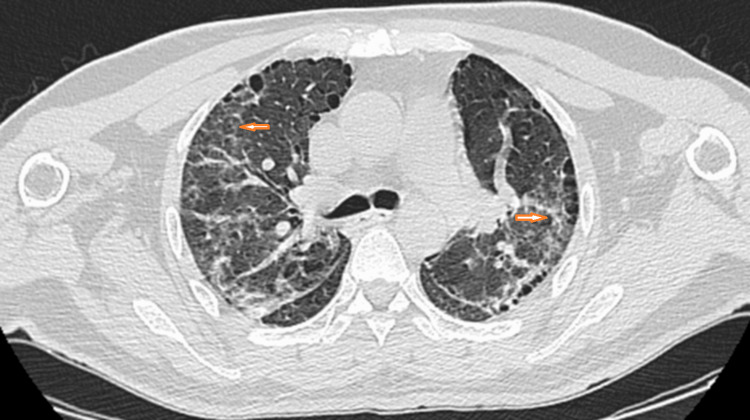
Axial CT scans of the chest with lung window settings, showing extensive ground-glass opacity.
